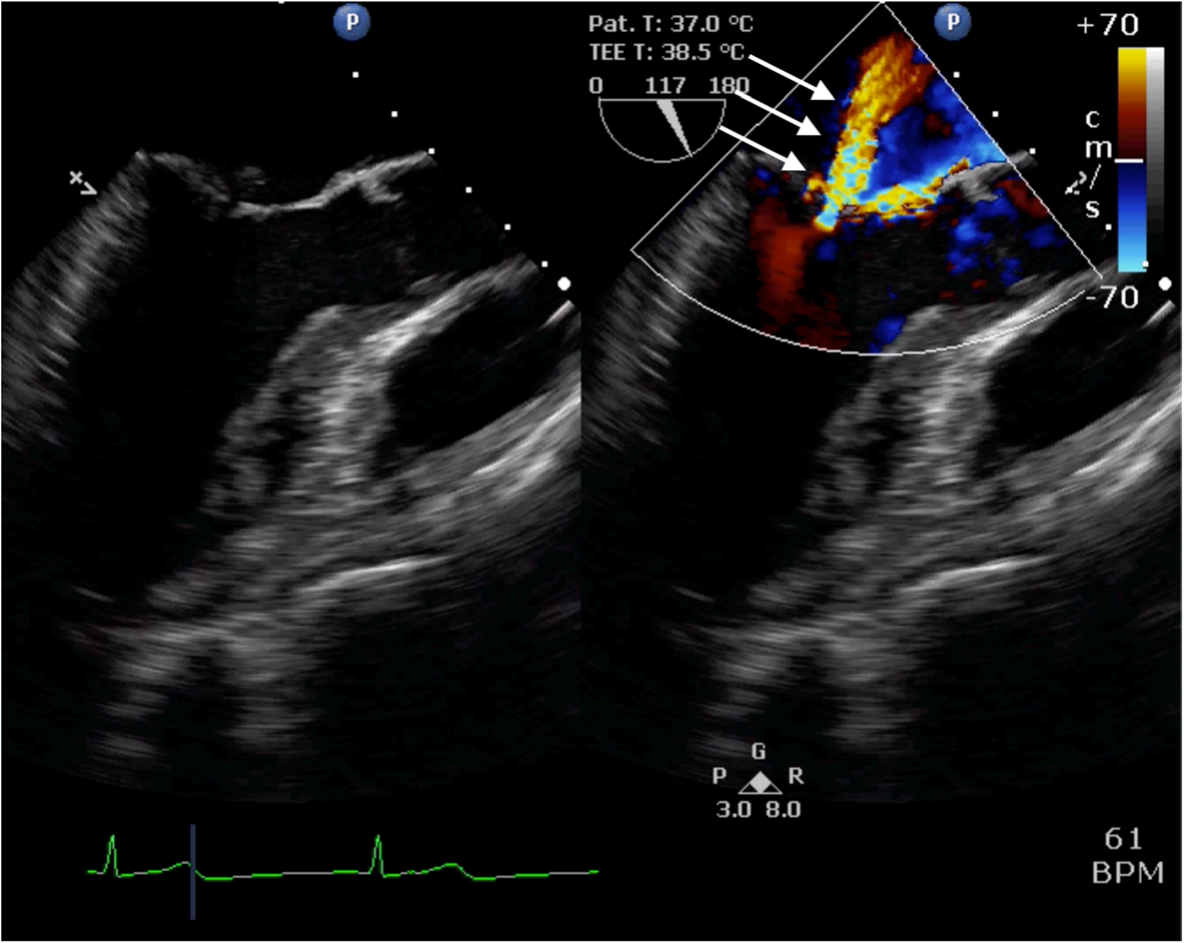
Clockwise rotation of probe in the mid-esophageal long-axis view shows normal configuration of the mitral leaflets (A3-P3), however, a color Doppler image reveals a central mitral valve regurgitation jet with unknown etiology (white arrow)
